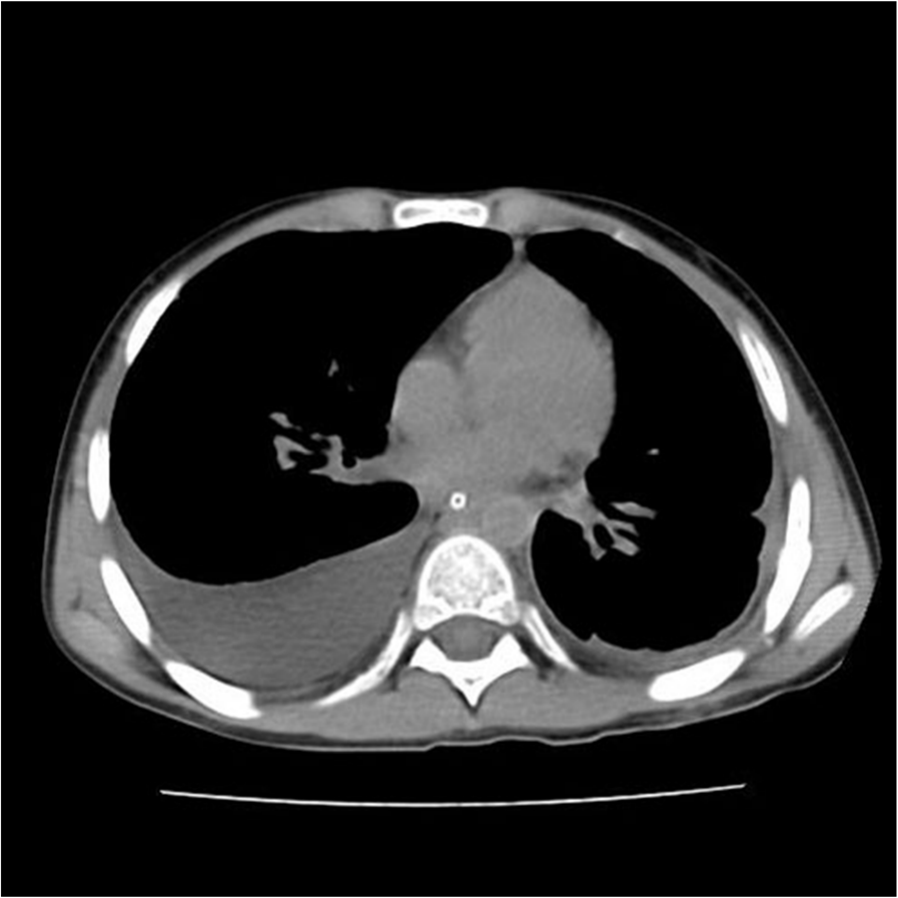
Pre-LAG CT of a nine-year-old boy had bilateral chylothorax after thoracic duct ligation.
